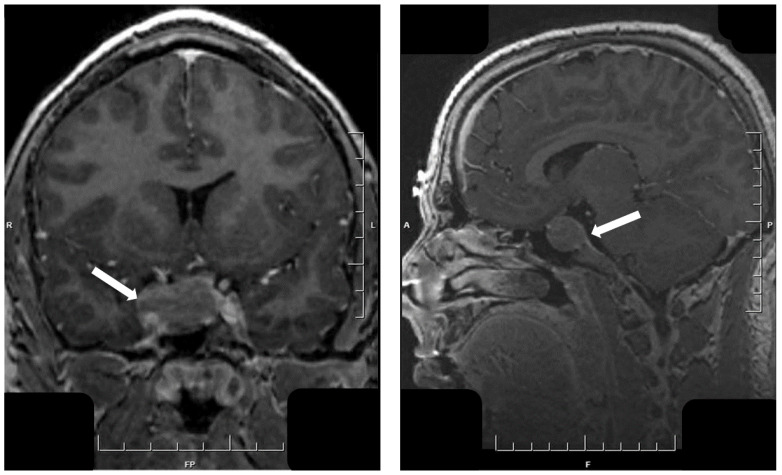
Magnetic resonance imaging of acidophil stem cell tumor. The large tumor that fills the sella turcica and extends into the right cavernous sinus (left, arrow) and characteristically downwards into the sphenoid sinus (right, arrow), was associated with hyperprolactinemia but not proportional to the tumor size; biochemical assessment documented mild elevation of IGF-1.
